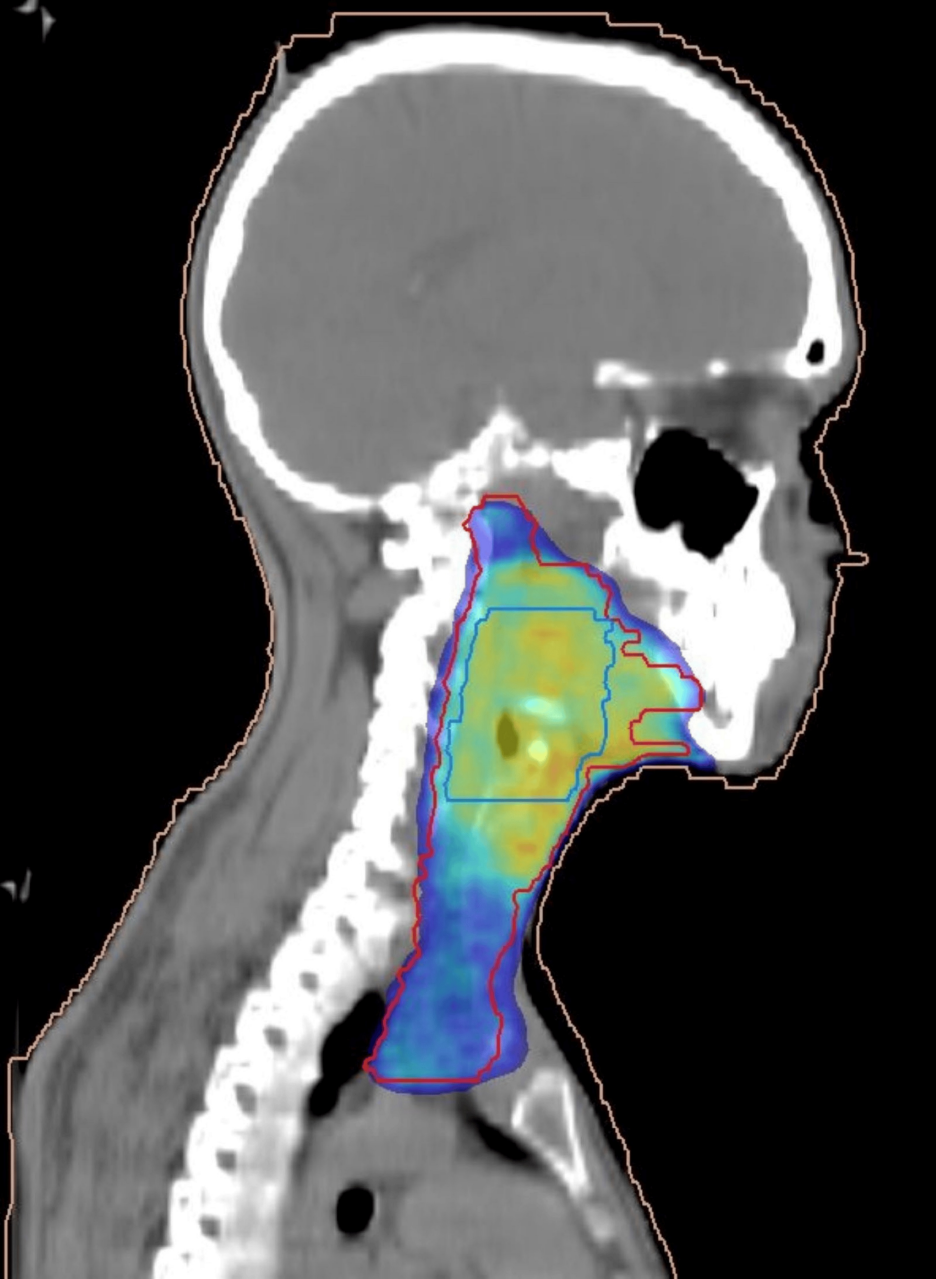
Sagittal CT slice of the same plan. The isodose wash demonstrates adequate target coverage with sharp dose fall-off to protect the spinal cord and surrounding normal structures.
